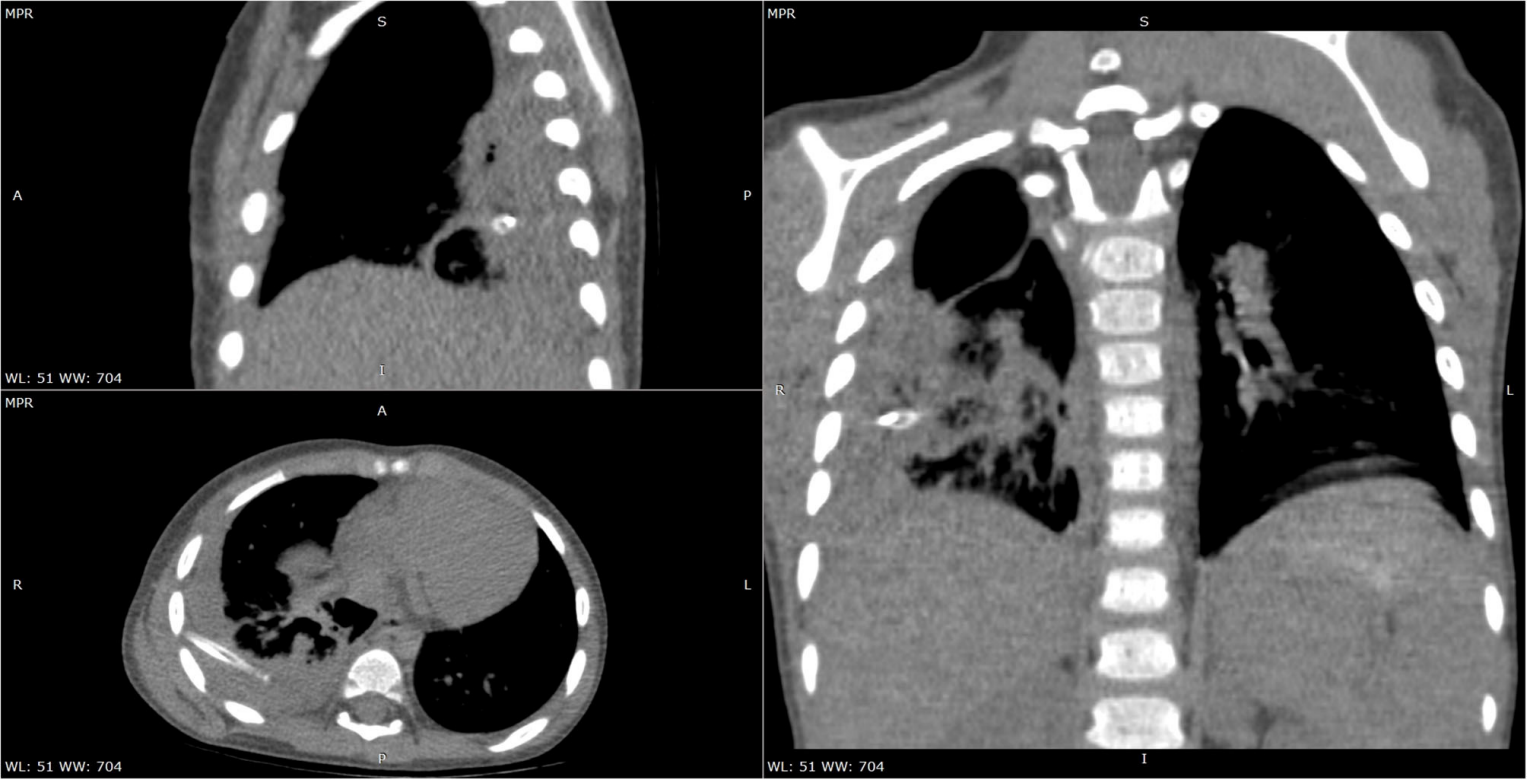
Chest CT scan—multiplanar reconstructions soft tissue window-encapsulated pleural effusion with drain tube.
